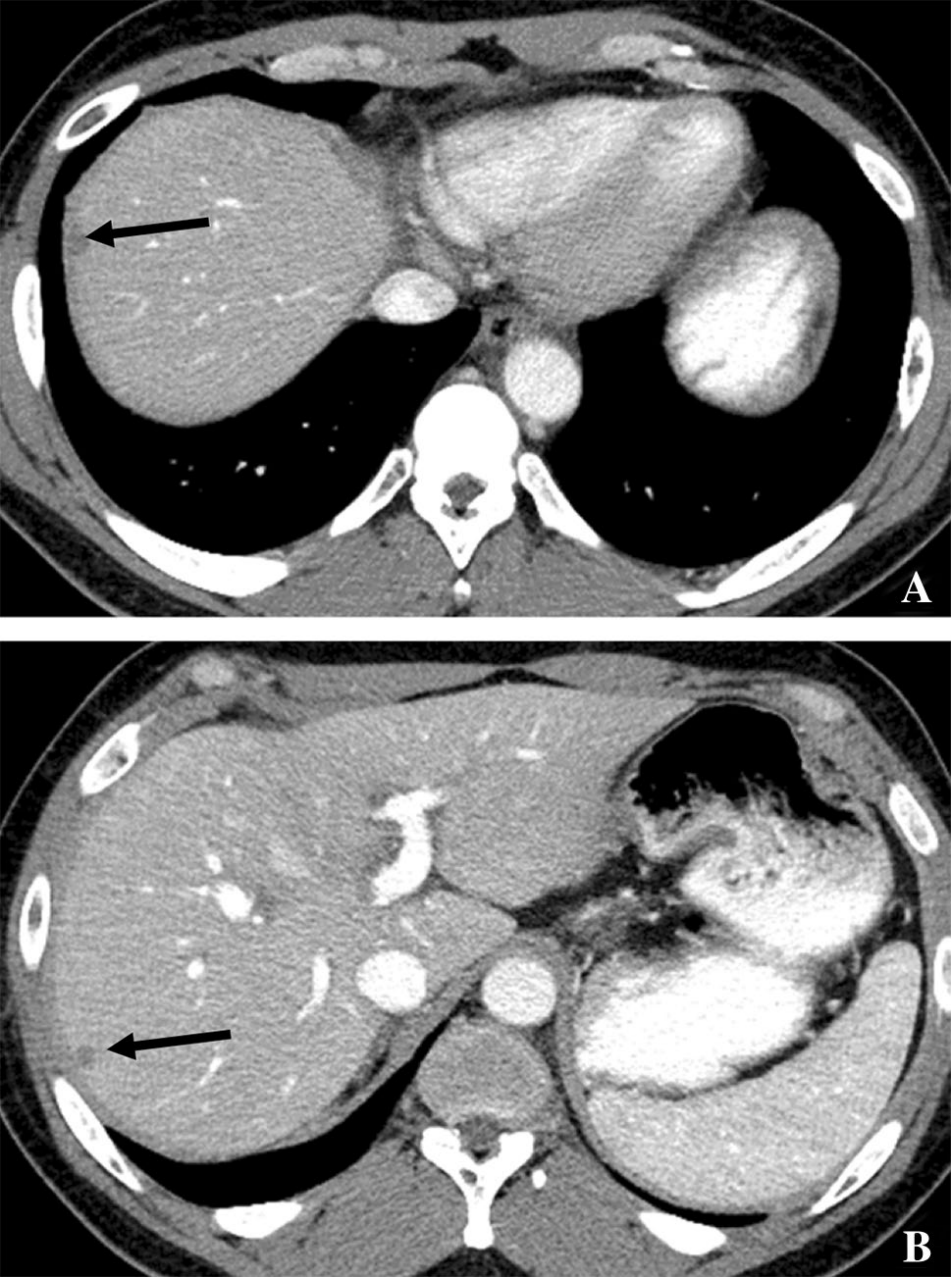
48-year-old man with KS and HIV. Contrast-enhanced axial CT A–B shows subcapsular low-attenuation lesions in the liver (arrows)
